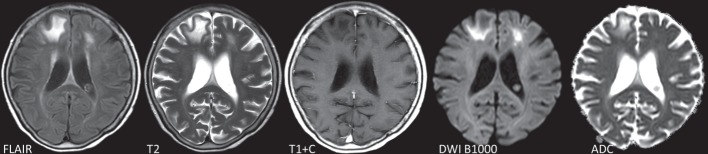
MRI brain showing white matter lesions in both frontal lobes affecting the deep and juxtacortical white matter. The lesions show a spreading along the white matter tract entering the contralateral hemisphere via the genu anterius of the corpus callosum.
